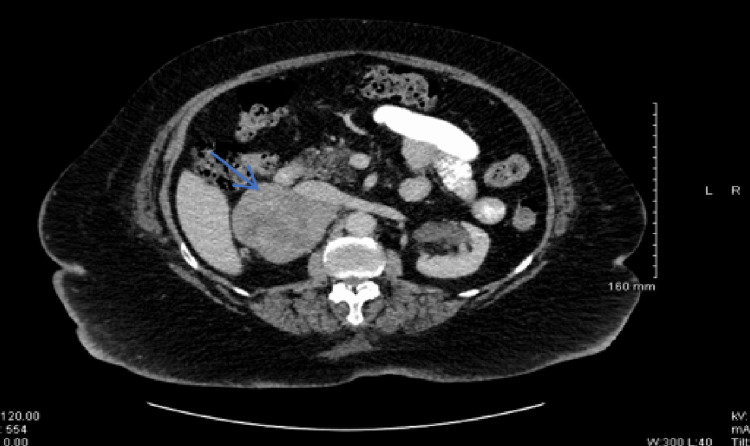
CT scan confirming the right adrenal mass (blue arrow)
